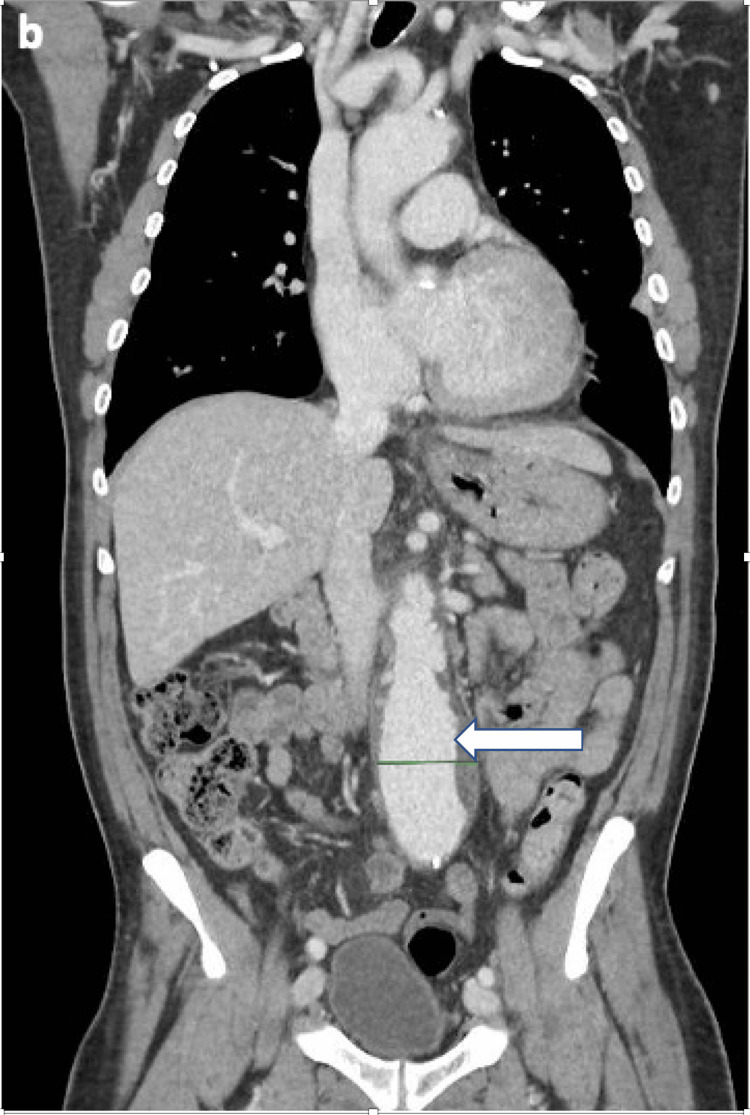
Axial CT aortogram image This single axial image from the November 2024 study shows the infrarenal abdominal aortic aneurysm (AAA). The white arrow indicates the large, concentric mural thrombus. The complex nature of the thrombus and vessel wall is consistent with the presence of atheromatous disease and penetrating ulcers as described in the formal radiology report, which are a known source for spontaneous cholesterol embolization. The lumen of the aneurysm is enhanced by intravascular contrast.
